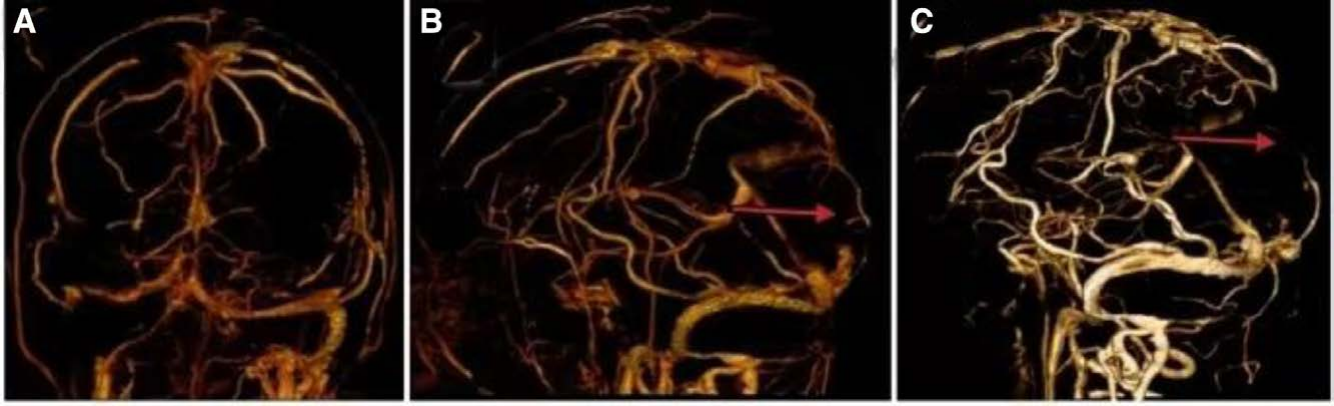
Computed tomographic venography (CTV) indicated that no contrast filling in the superior sagittal sinus, CVST was considered, the right transverse sinus and sigmoid sinus were small and locally discontinuous, anatomical variants were considered (A, B). The follow-up cerebral CTV showed no contrast-filled superior sagittal sinus (C). CVST = Cerebral venous sinus thrombosis.
